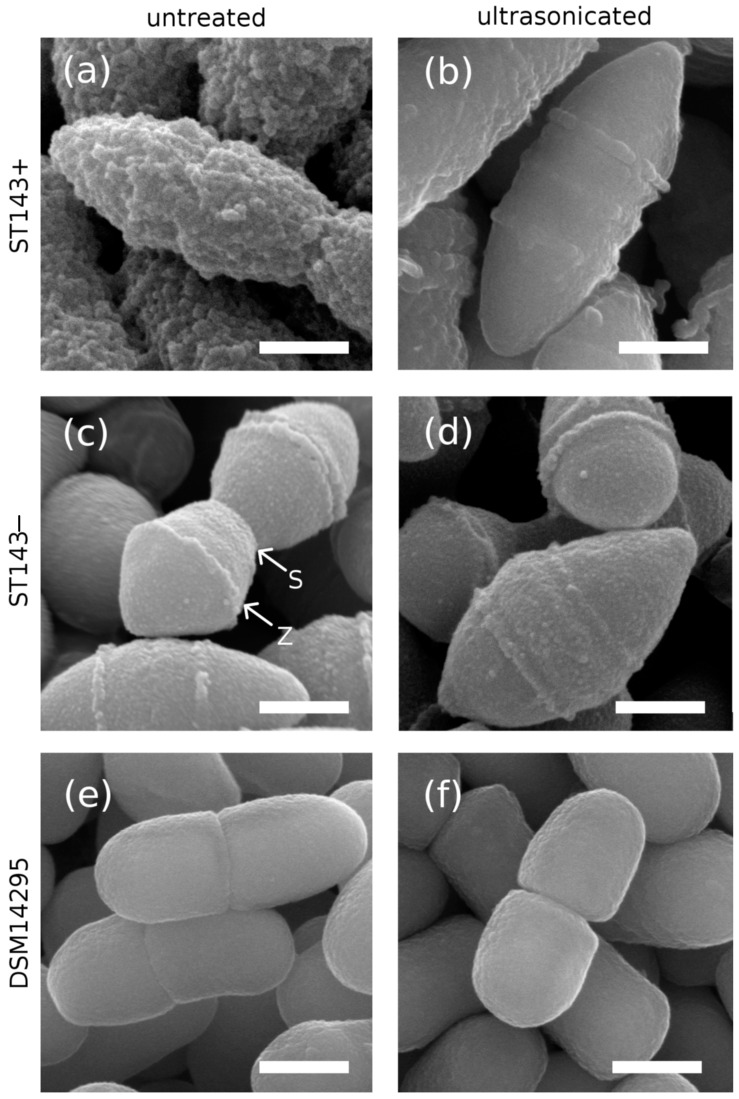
Comparison of the cells of *S. thermophilus* ST143+ (a,b), ST143− (c,d), and *W. cibaria* DSM14295 (e,f) before (left) and after (right) ultrasonic treatment for CPS removal as demonstrated by SEM images. Z: Z-ring; S: septum; scale bar: 500 nm.
